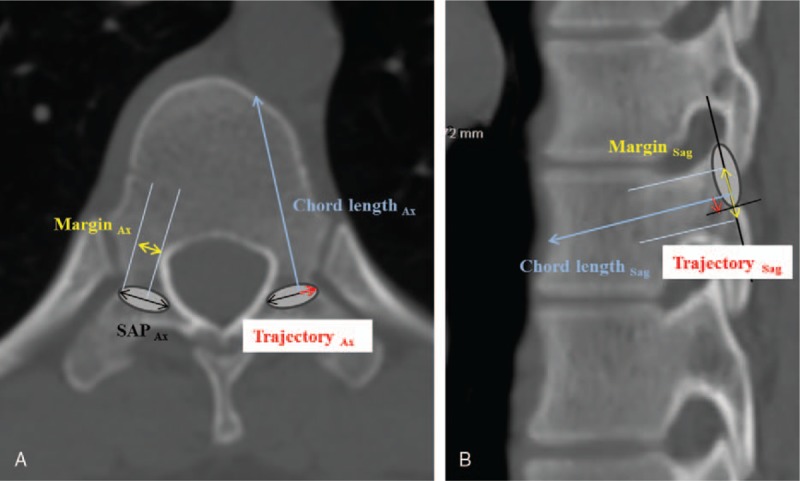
The axial (A) and sagittal (B) CT image showing the radiological parameters (yellow arrowed line: Margin_Ax_ and Margin_Sag_, blue arrowed line: CL_Ax_ and CL_Sag_, and red arrowed line: Trajectory_Ax_ and Trajectory_Sag_). CT = computed tomography.
